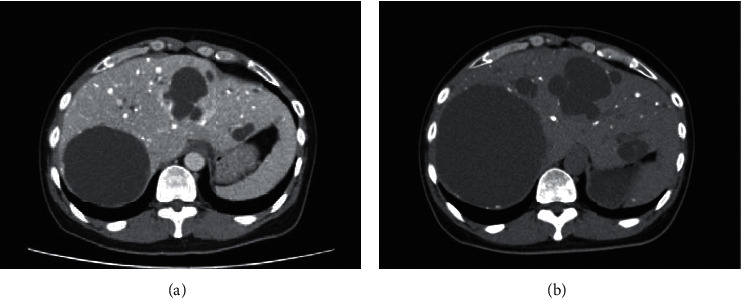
Computed tomography revealed that the liver cysts were increased in size after vandetanib treatment. (a) At 42 months after vandetanib treatment initiation. (b) At 48 months after vandetanib treatment initiation, abdominal distension developed.
